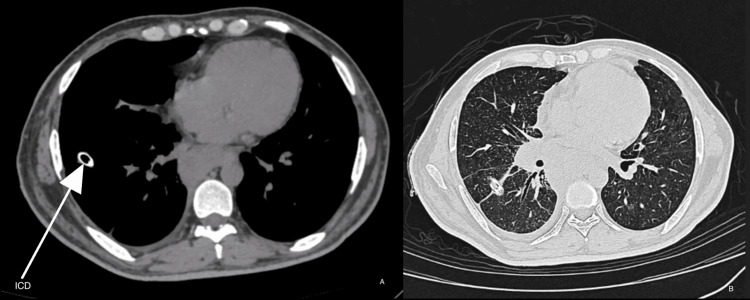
HRCT chest showing ICD in situ HRCT: High-resolution computed tomography, ICD: Intercostal drain, A: Mediastinal Window, B: Lung Window
